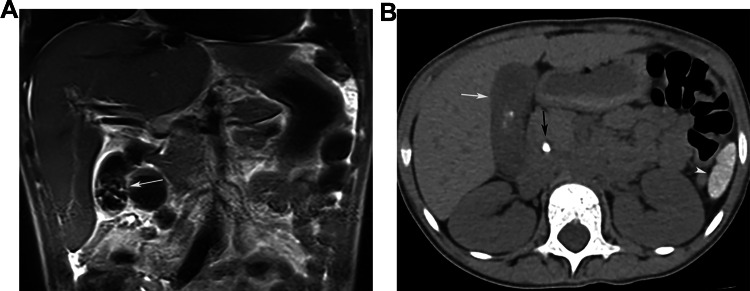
a, b Biliary manifestations of sickle cell disease. a Coronal T2-weighted MRI of a 36-year-old male presenting with jaundice, diarrhea, and vomiting, followed by progressive disorientation over two days. The image shows a gallbladder filled with gallstones (arrow). b Non-contrast CT scan of a 35-year-old female presenting with fever, acute abdominal pain, and nausea. The image demonstrates a thick-walled gallbladder containing calculi, consistent with cholecystitis (white arrow). A calculus is also visualized in the common bile duct, indicating choledocholithiasis (black arrow). Evidence of autosplenectomy is noted (arrowhead)
